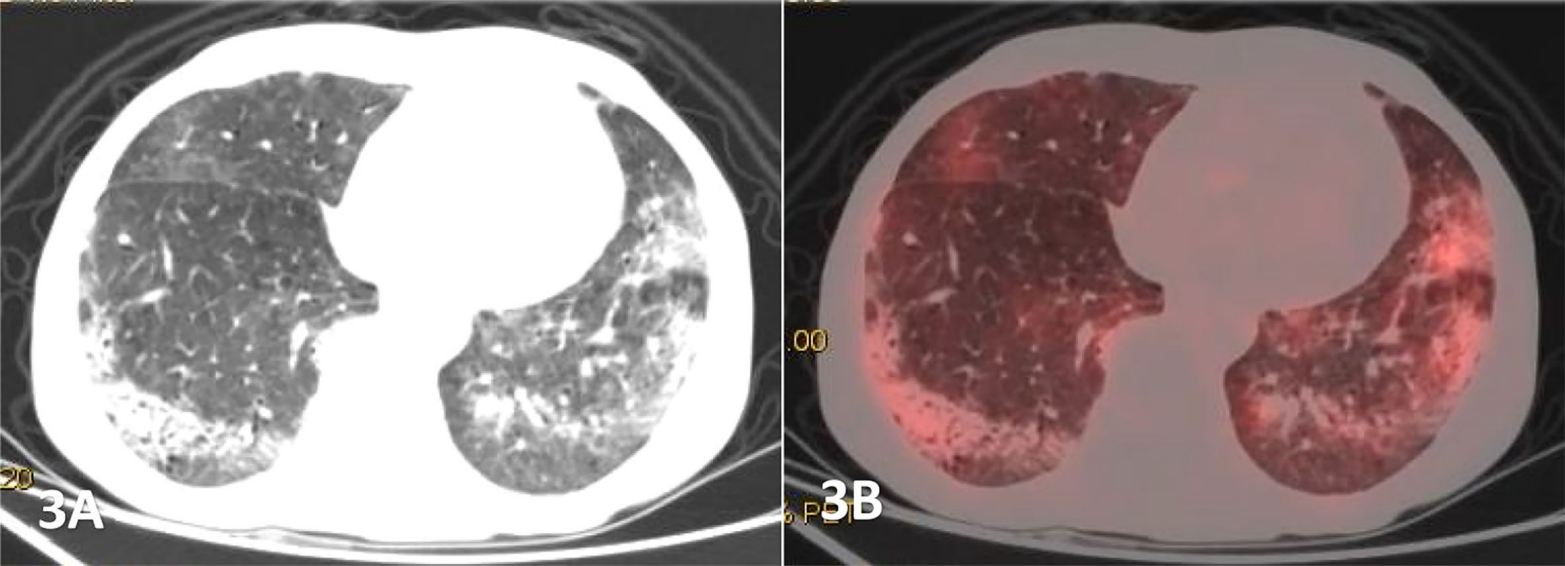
Male, 68 years old, (A) was transverse CT, and (B) was PET/CT fusion, Diffuse patchy, grid-like increased density shadow was seen in both lungs, which was obvious in two lower lungs with unclear margins. The FDG uptake of the lesions were increased, with a SUVmax of 4.27,and pathological findings of percutaneous lung biopsy were MALT.
